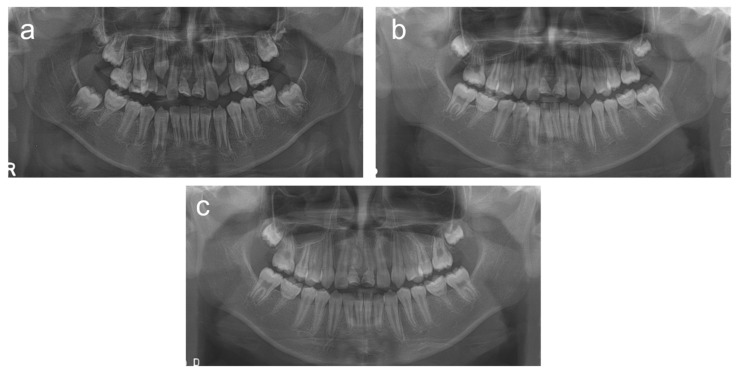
Panoramic radiographs of patient 2. (a) Panoramic radiograph at age 9 years 7 months. (b) Panoramic radiograph at age 10 years 10 months. (c) Panoramic radiograph at age 11 years 8 months.
